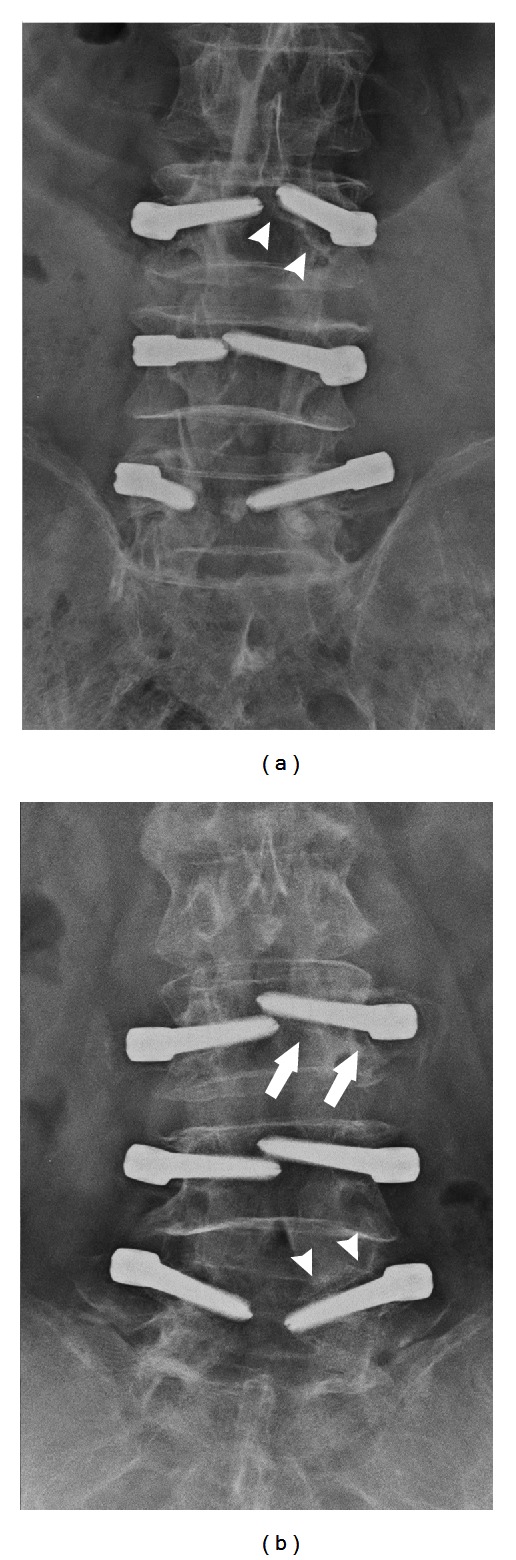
Anteroposterior radiographs. (a) A 61-year-old female who underwent Dynesys stabilization at 12-month postoperation. The double halo sign indicated loosening of the left L3 pedicle screw. (b) A 75-year-old male who underwent Dynesys stabilization, at 2-year postoperation. The double halo sign indicated screw loosening of the left L5 and the halo sign indicated screw loosening of the left L3 screws. Arrowhead, double halo sign; arrow, halo sign.
